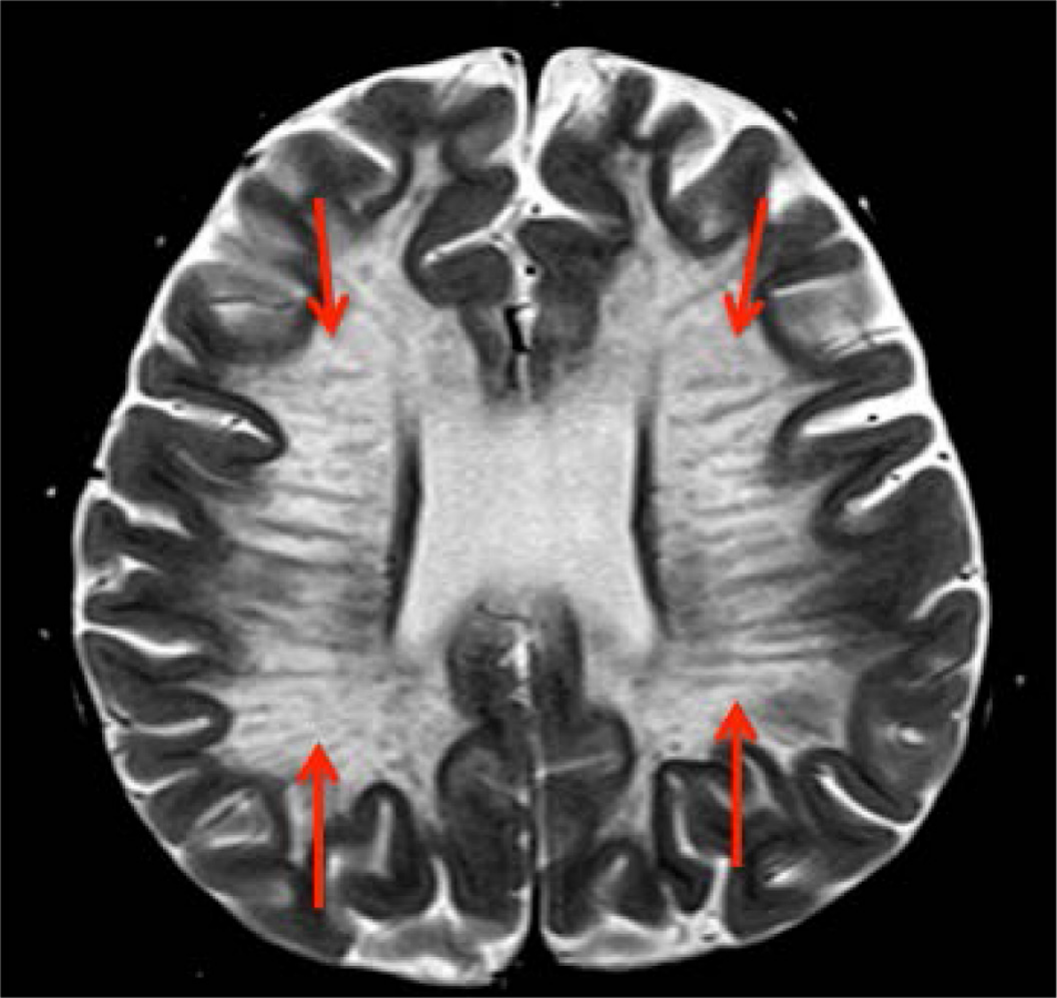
Magnetic resonance imaging of patient’s brain showing classic “Tigroid” or “Leopard Skin” pattern in the periventricular white matter on T2W images, consistent with metachromatic leukodystrophy (red arrows).
